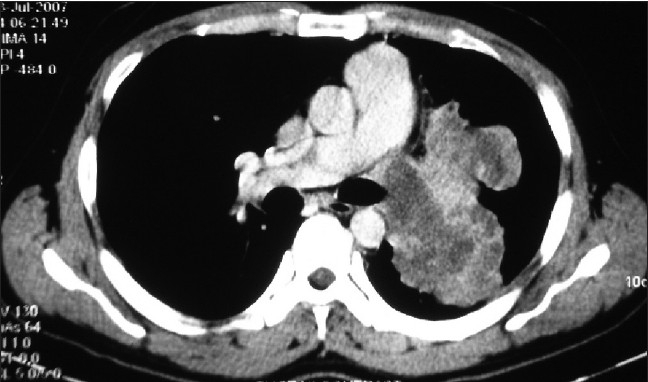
CT scan of the thorax showing large well defined lobulated, nonhomogenous mass extending up to the pleural surface
